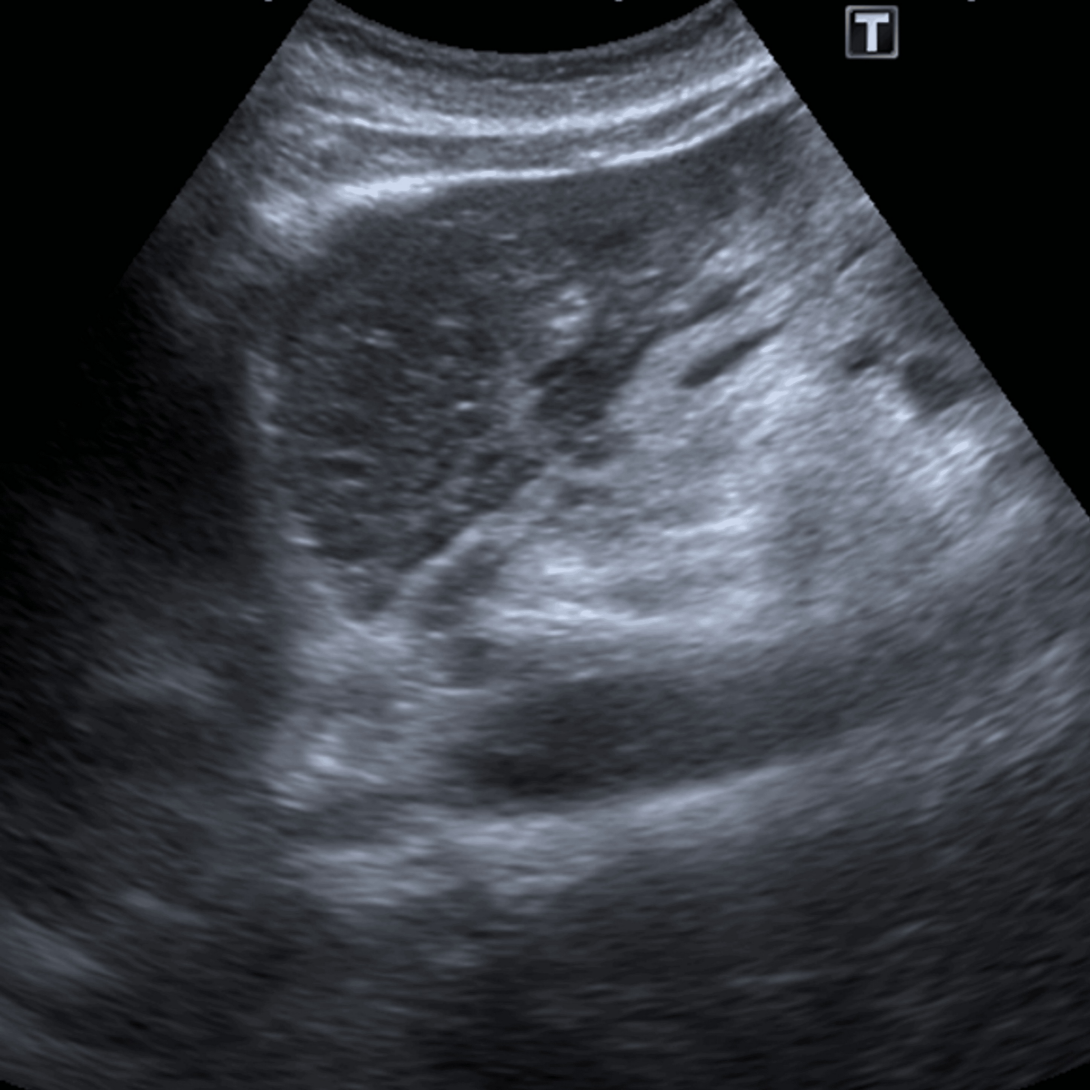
Abdominal ultrasound image of the patient’s globose liver
